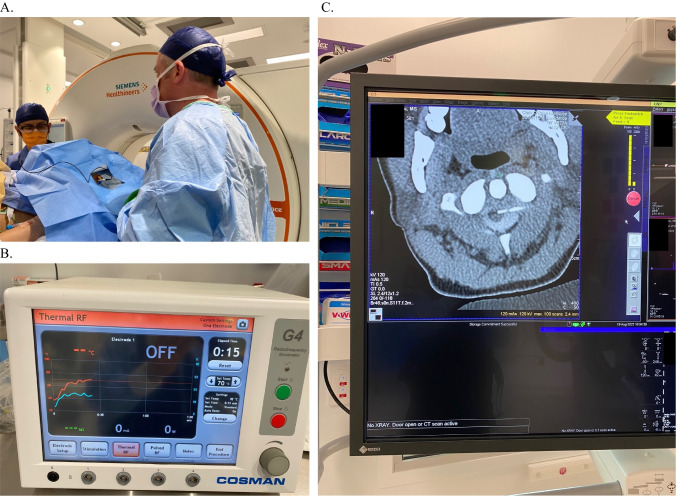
Percutaneous cordotomy procedure for treatment of right hip and leg pain caused by metastatic NSCLC (non-small cell lung cancer) in the pelvic region. A A needle with pulse generator is placed over left neck region for real-time sensory testing to precisely localise the area of pain with CT image guidance. B Radiofrequency device providing thermal lesioning of the spinothalamic fibers. C CT image inside theatre guiding needle placement through C1/2 vertebral space into targeted spinal cord
